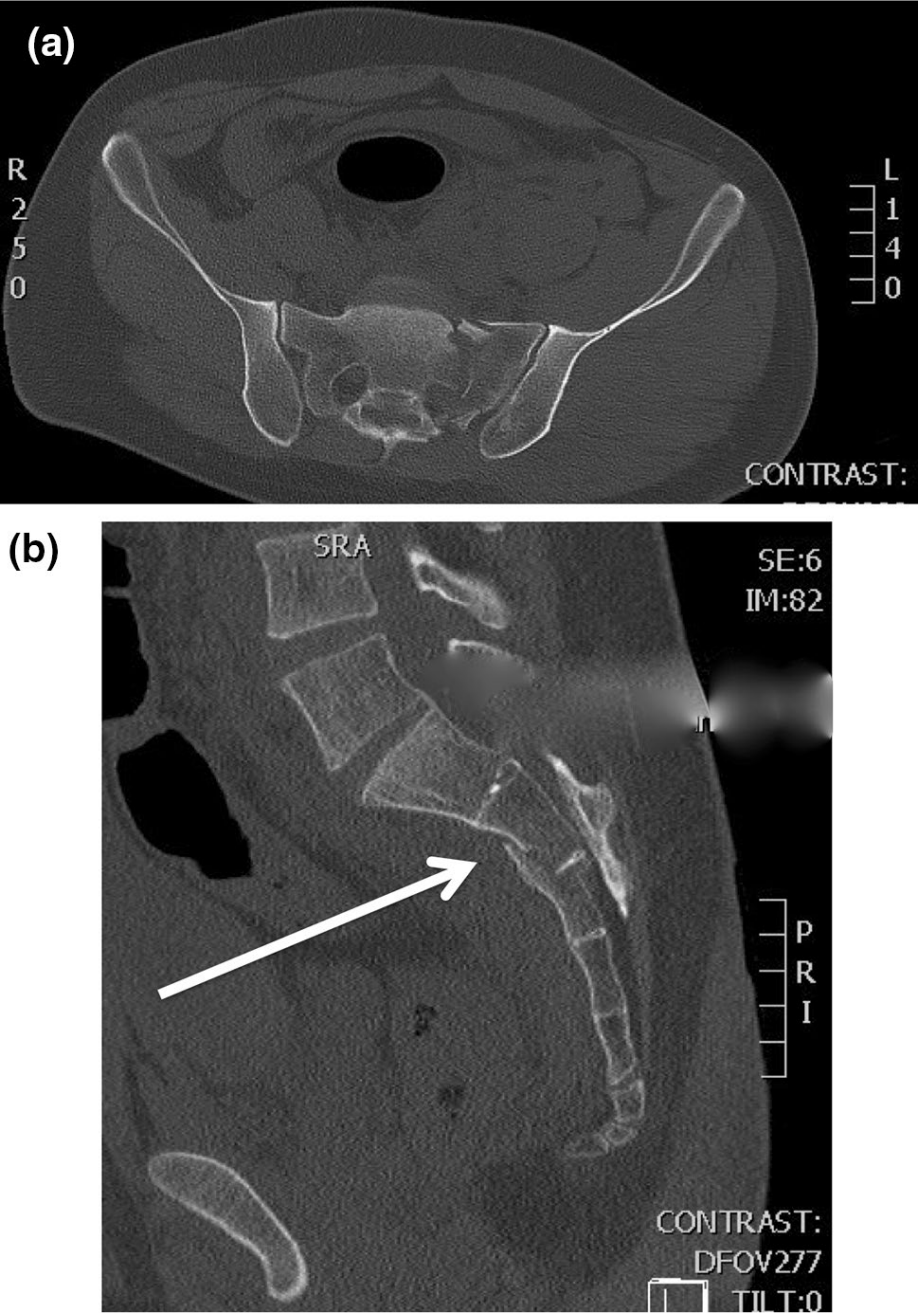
CT scans of all patients have been performed. a Demonstrates a comminuted sacral fracture. The sagittal reconstruction (b) shows the horizontal fracture line leading to rotational instability
